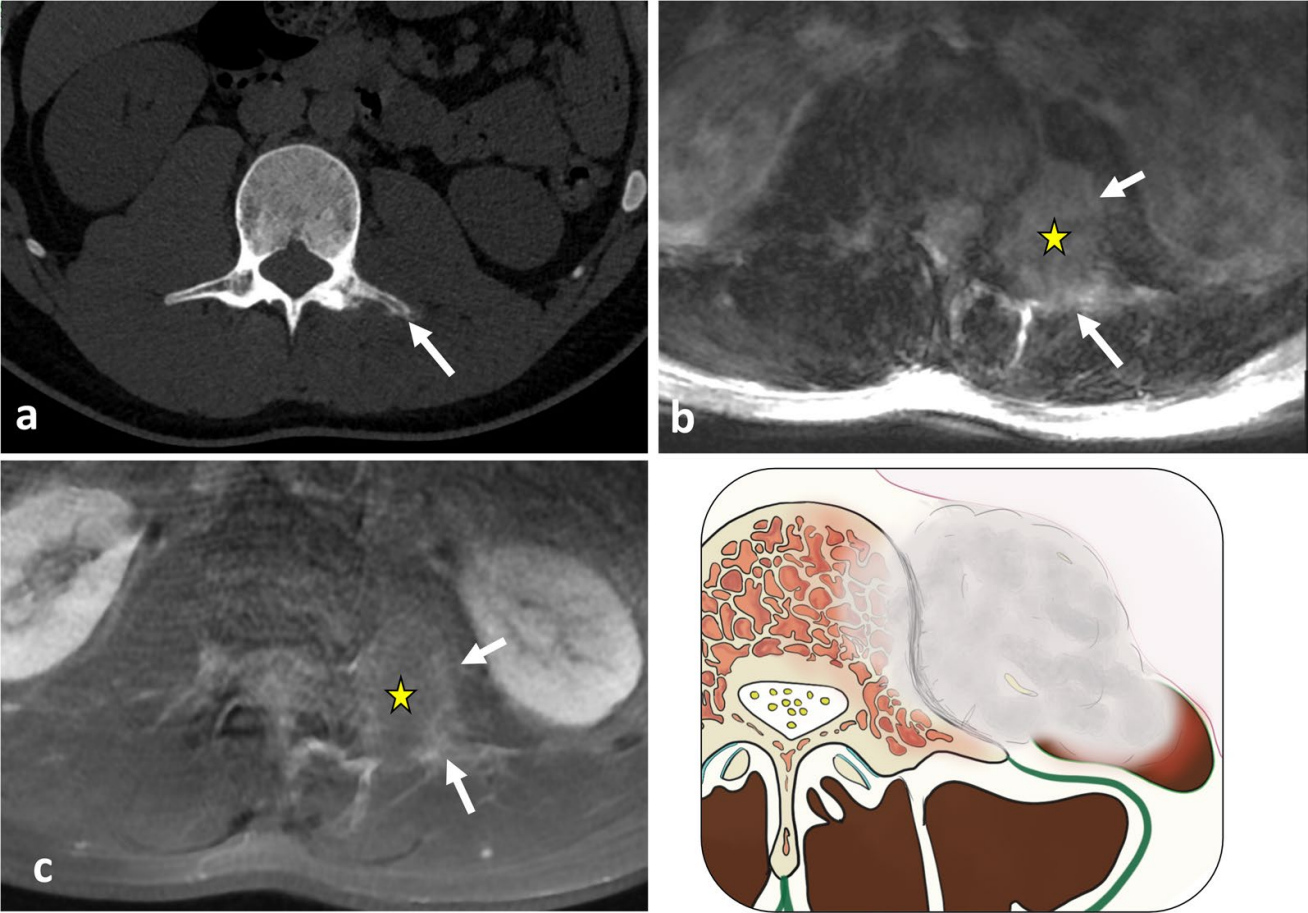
Ewing sarcoma* of L4 in an 18-year-old male with extreme low back pain and acute cauda equina syndrome. Axial CT reconstruction (a) demonstrates a moth-eaten lytic lesion of the transverse process with large paraspinal soft tissue component, best seen on axial T2WI (b) and axial contrast-enhanced, fat-suppressed T1WI (c). Yellow star shows the epicentre of the mass. Lying position in MRI tube caused intense spinal pain, which explains motion of the patient and motion artefacts
